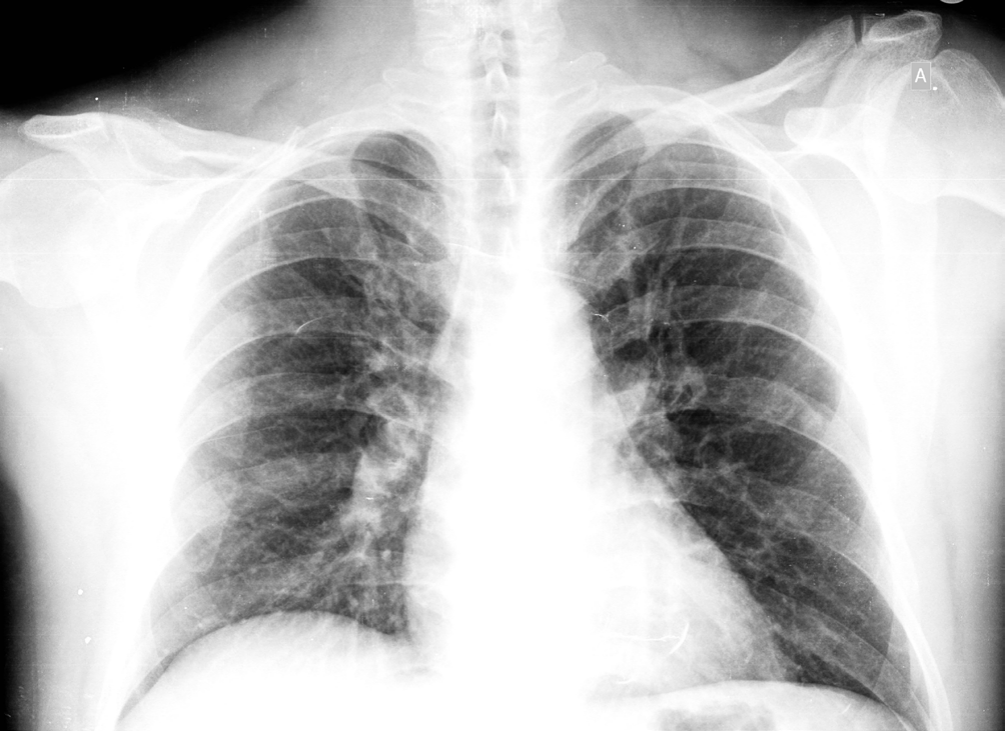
Chest x-ray on admission with no apparent findings.
